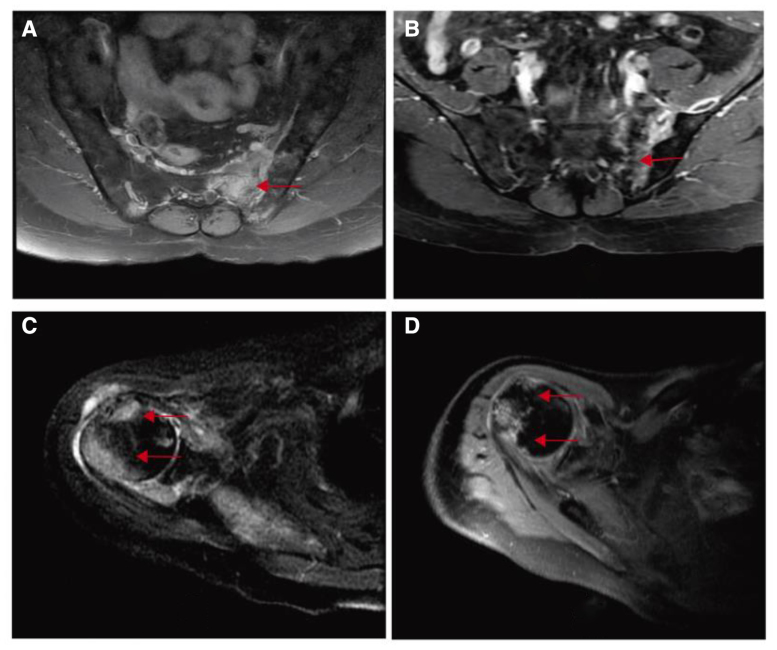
(A) On admission, pelvic MRI shows diffuse bony destruction beneath both sacroiliac joint surfaces, in the left iliac bone, and in the lumbosacral vertebrae, accompanied by localized soft-tissue mass formation at the left sacroiliac joint. (B) After 5 months of antituberculosis treatment, repeat pelvic MRI shows improvement of the bilateral sacroiliac joint surfaces and iliac bone compared with before. (C) On admission, MRI of the right upper arm demonstrates a long T1/long T2 signal in the right humeral head and neck, accompanied by surrounding tissue thickening and edema. (D) After 4 months of antituberculosis treatment, MRI of the right humeral head revealed abnormal bone signal intensity with surrounding tissue thickening and edema, showing improvement compared with previous findings.
